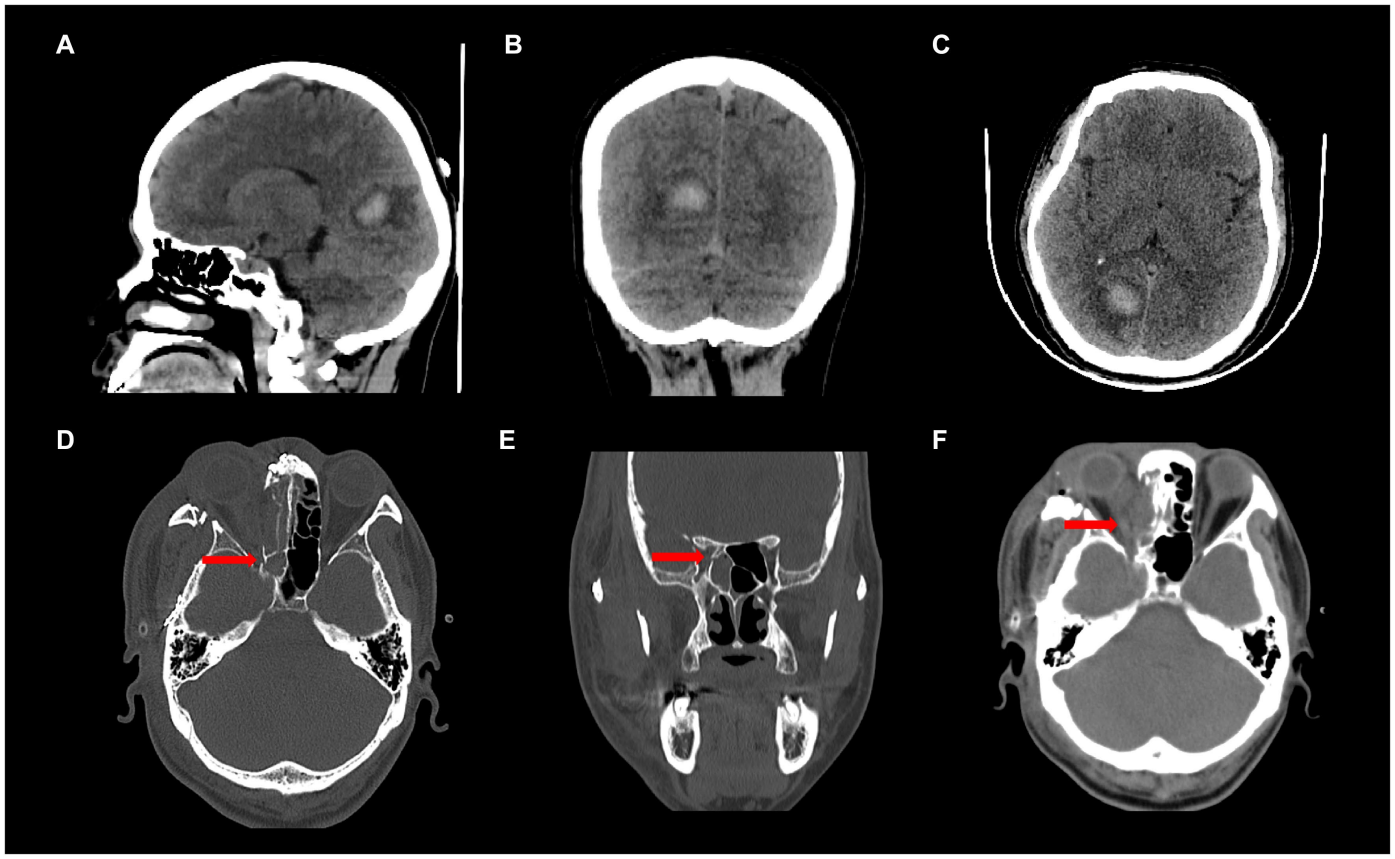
(A-C) Patient 1, CT findings showing right occipital lobe hemorrhage; (D-F) Patient 2, CT findings showing a fracture of the optic canal and swelling of the optic nerve on the right side.
